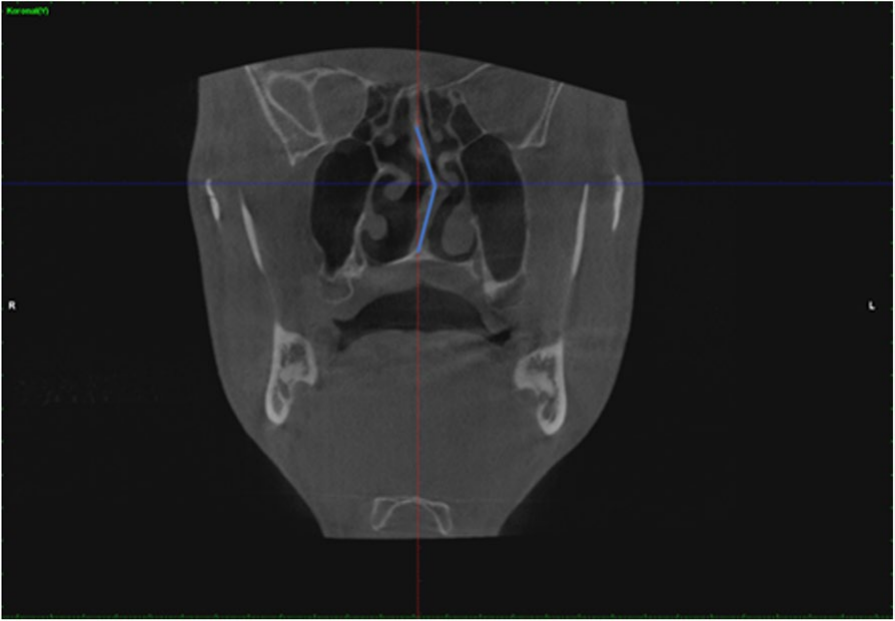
Determination of nasal septum deviation in the coronal CBCT images
